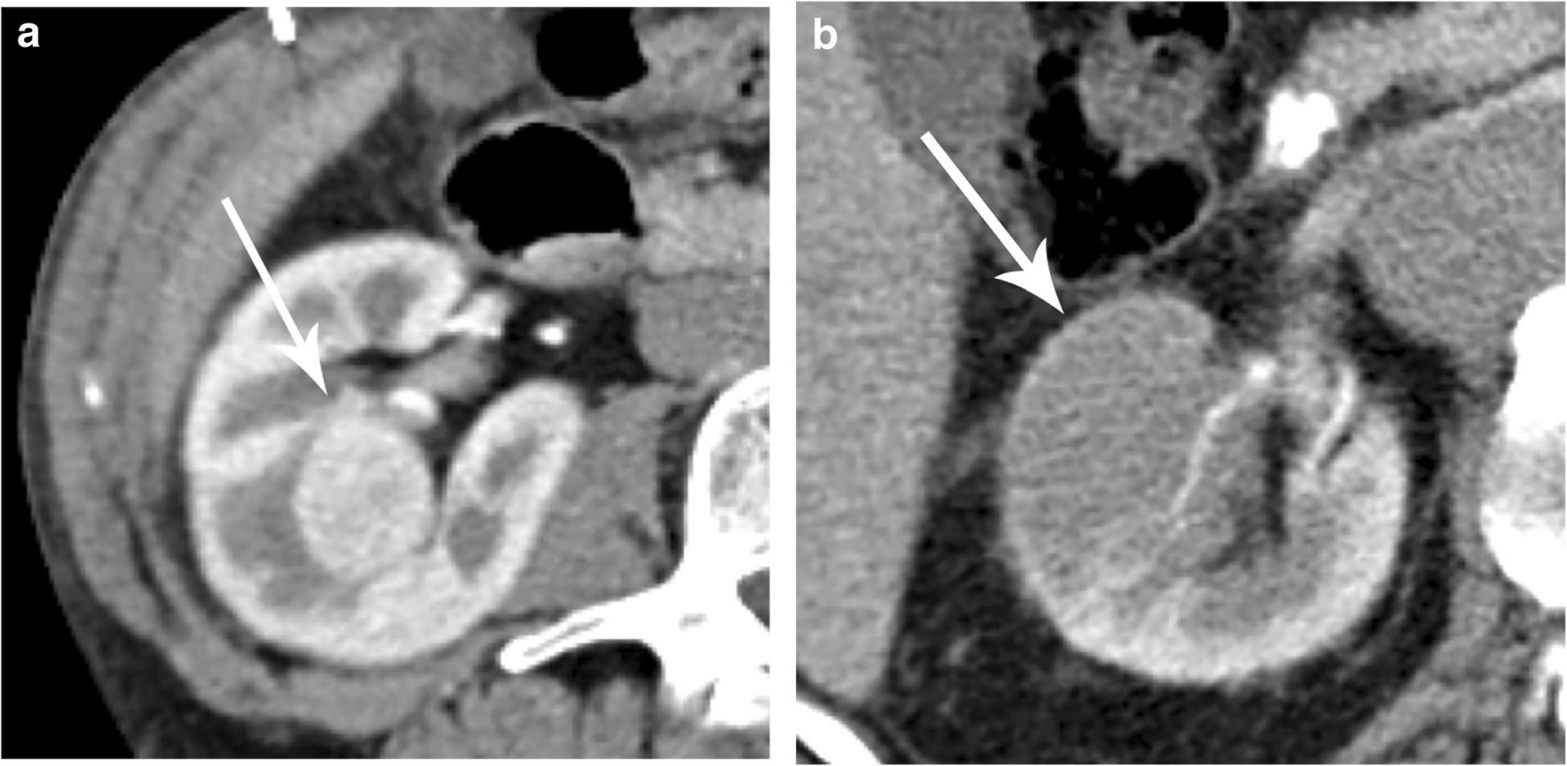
Solid renal masses in two elderly patients with regular and irregular borders. a Axial contrast-enhanced CT in the arterial phase shows a hyperdense right renal mass with smooth margins and regular shape (arrow) which, if indicated, can be managed with active surveillance. b Axial contrast-enhanced CT scan in the nephrographic phase depicting a hypodense renal mass with irregular borders (arrow). Active surveillance is not indicated in this case
